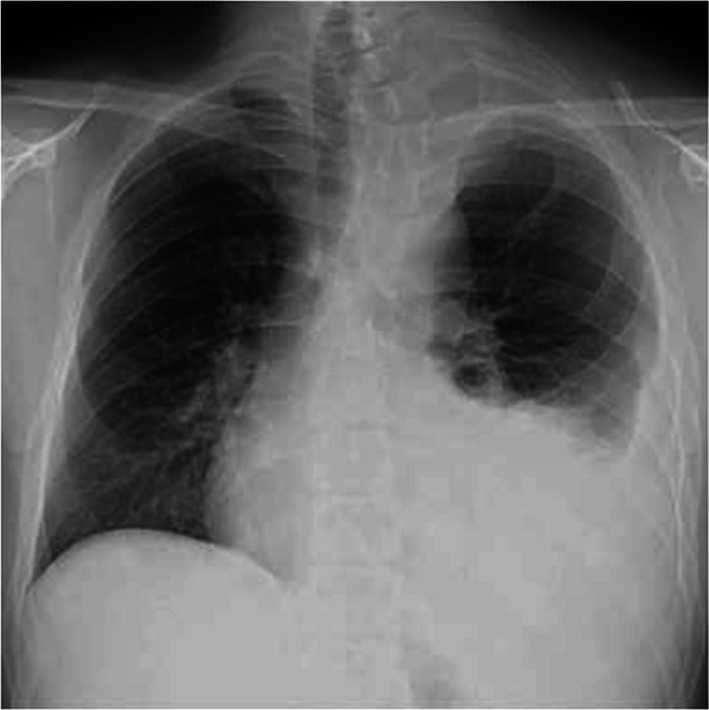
Preoperative chest radiograph. Chest radiography shows a left-sided pleural effusion and an opacity at the apex of the left lung
